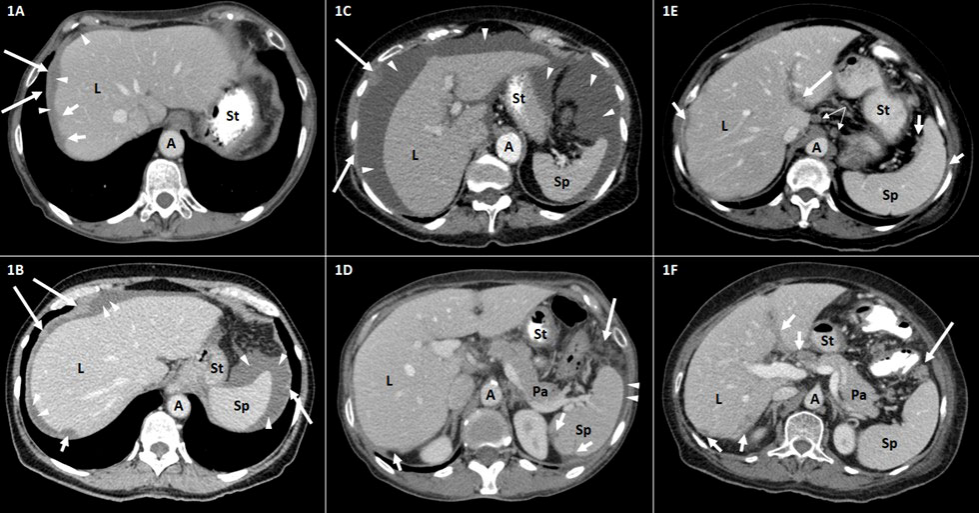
Representative CT findings in the upper abdomen in patients with advanced ovarian carcinoma FIGO stage III–IV. Axial contrast-enhanced CT scans of the upper abdomen (A–F). A, aorta; L, liver; Pa, pancreas; Sp, spleen; St, stomach. (A, B) Tumor implants of the diaphragm (long arrows) and the liver (short arrows). The thickening of the right hemidiaphragm (long arrows) can be distinguished from surrounding perihepatic ascites (arrowheads). The surface tumor deposit at the dome of the liver (short arrows) causes scalloping of the lateral (A) and posterior (B) liver surface. (C) Large amount of ascites (arrowheads) in the upper abdomen. Peritoneal knotty implants (long arrows) are shown. (D) Tumor scalloping (short arrows) of the posterior surface of the spleen and the liver. Small amount of perisplenic ascites (arrowheads). Fat tissue stranding and tumor nodularity (long arrow) are seen in the fat adjacent to the splenic flexure of the colon. (E) Tumor implants (long arrow) along the hepatogastric ligament. Peritoneal thickening and tumor scalloping of the surface of the spleen and the liver (short arrows). Round suprarenal lymph nodes (narrow arrows). (F) Tumor implants (short arrows) in the porta hepatis along the falciform ligament and of the posterior liver surface. Tumor nodule (long arrow) is seen in the fat adjacent to the splenic flexure of the colon. FIGO, International Federation of Gynecology and Obstetrics.
